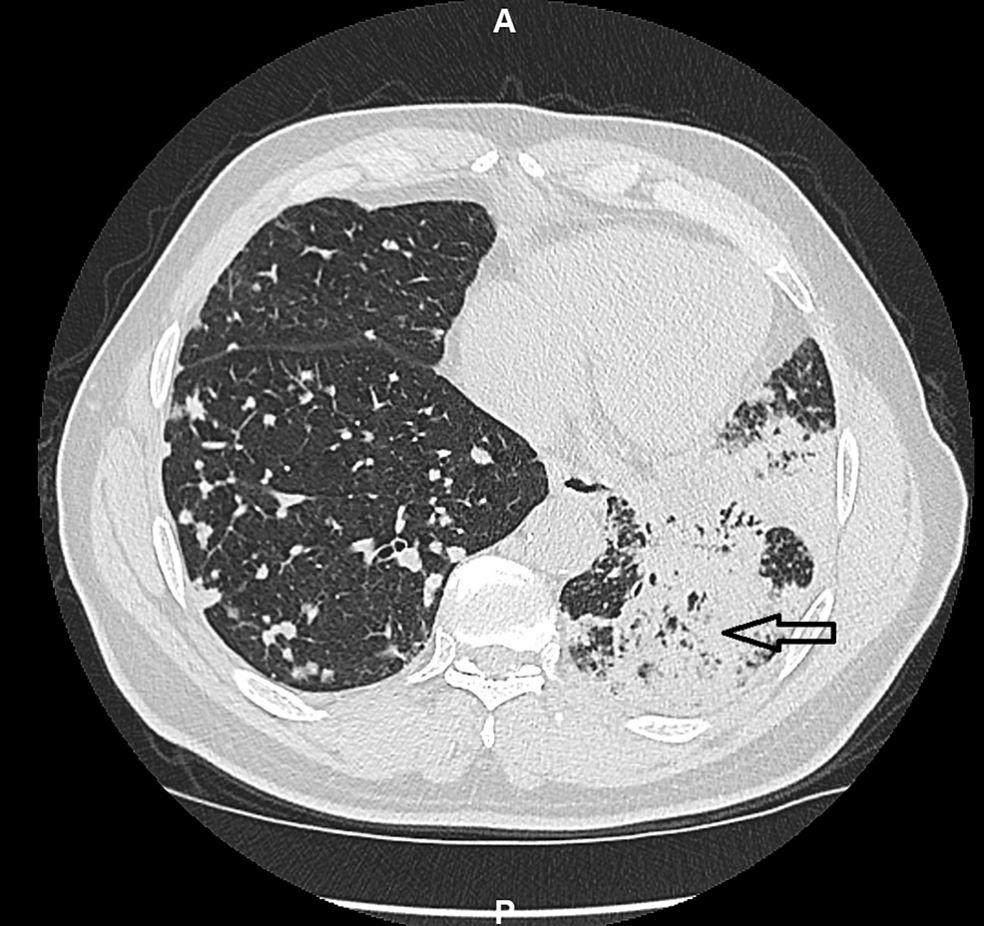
Axial section of CT chest shows extensive pneumonic infiltrates in the left lower lobe.
